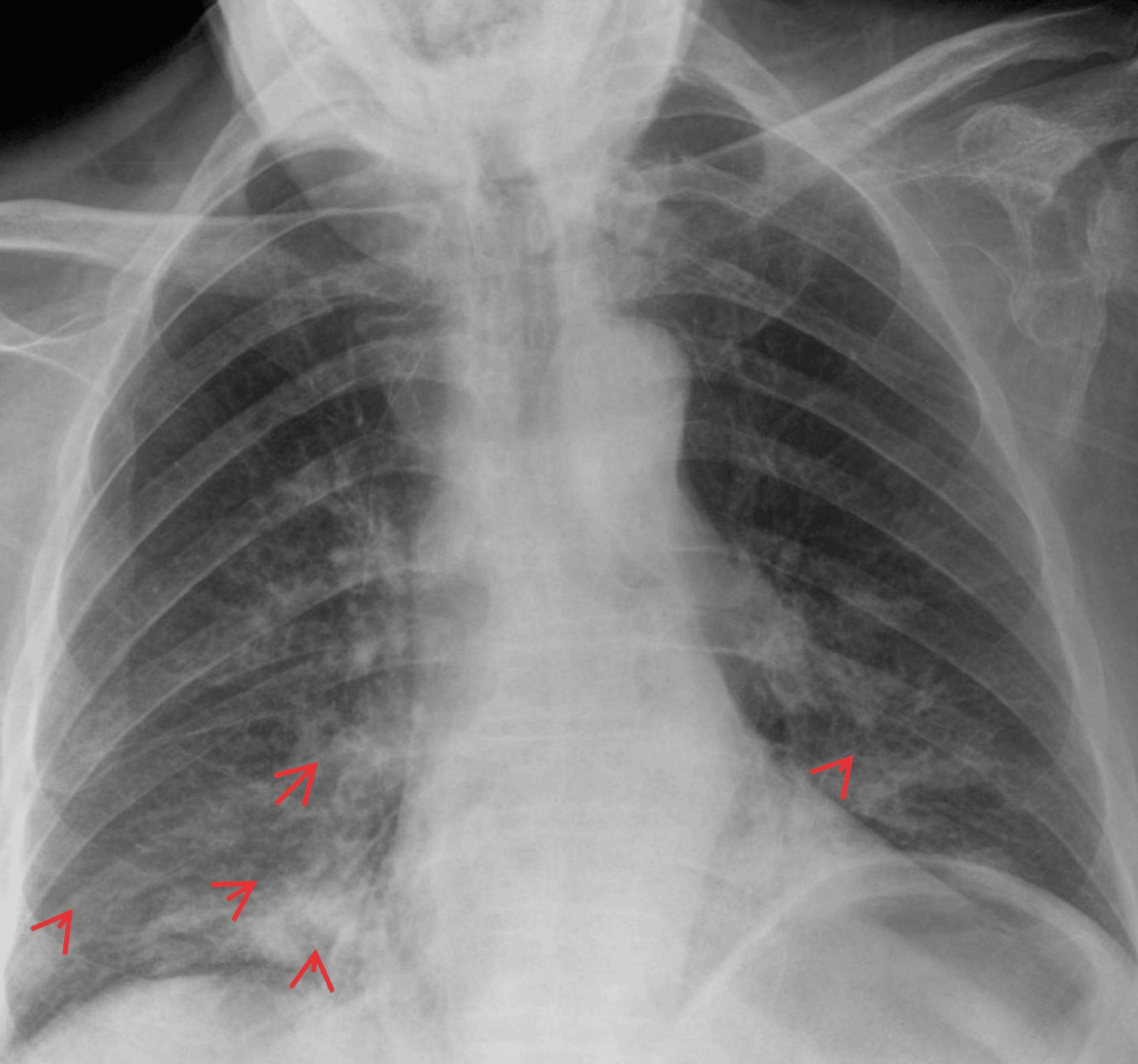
Chest X-ray showing bilateral lower zone opacities and areas of increased density (red arrows)
